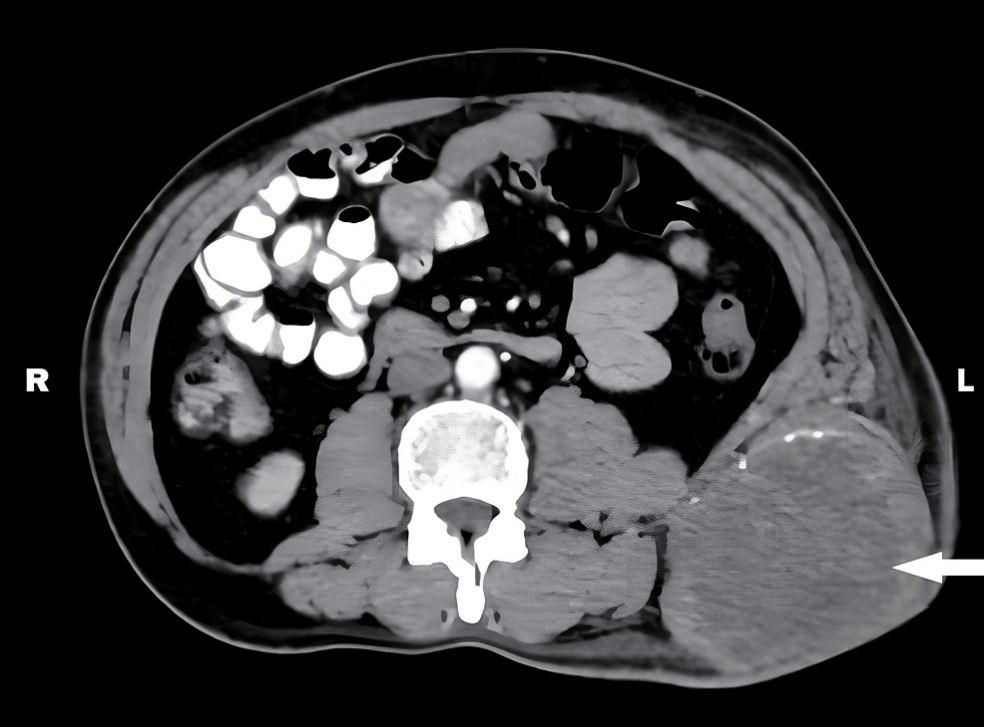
CECT scan of the abdomen and pelvis A contrast-enhanced CT (CECT) scan of the abdomen and pelvis revealed a lesion in the anterior abdominal wall, as shown with the white arrow.
